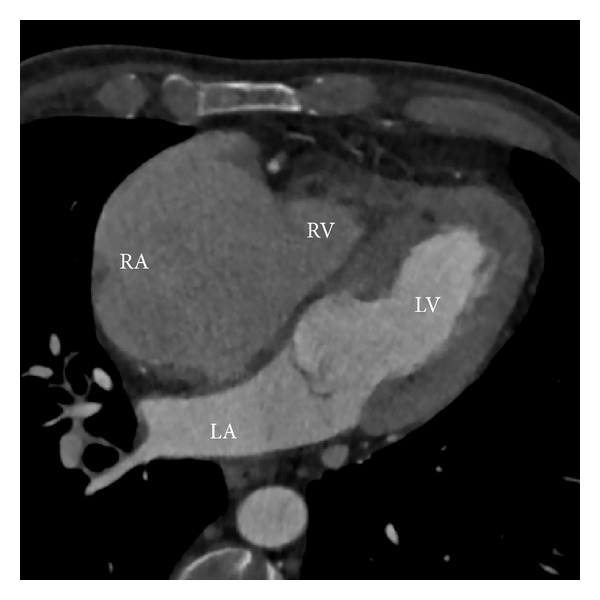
Cardiac CT five years after the MRI scan. The right atrial cavity is now markedly enlarged, and the right ventricular cavity is almost obliterated. The left-sided cavities appear normal. RA: right atrium; RV: right ventricle; LA: left atrium; LV: left ventricle.
